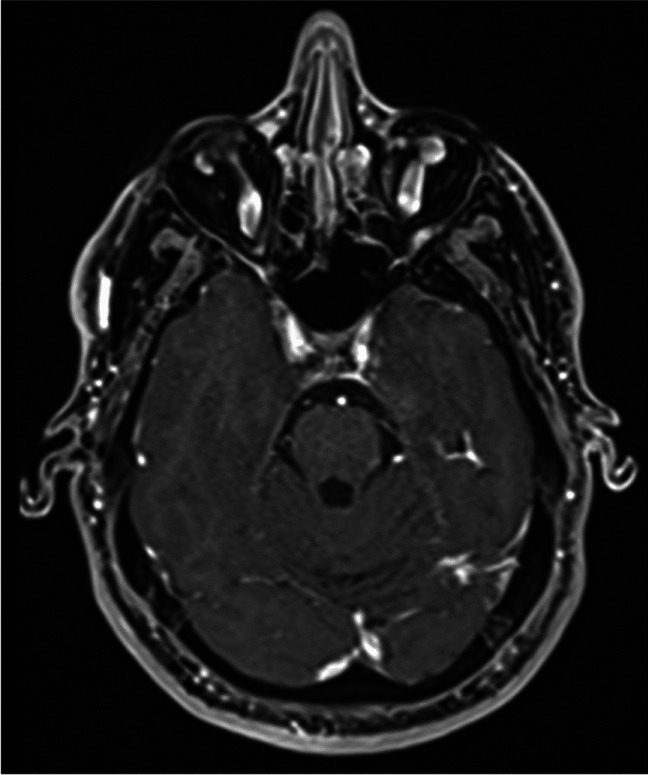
Follow-up MRI at six months showing complete asportation of the tumor and re-epithelization of the skull base
